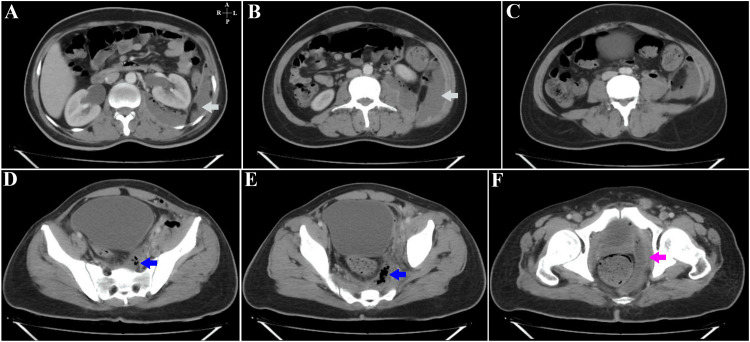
Patient 1- Contrast-enhanced CT images of the abdomen in the axial plane Figure 3A – At the level of the L3-L4 intervertebral disc space, showing a peripherally enhancing collection (cyan arrow) in the posterior, left lateral extra-peritoneal space, extending posterior to the descending colon and the left kidney. Figure 3B – Through the body of the L4 vertebra, an extension of the same collection (cyan arrow) is seen with fascial thickening and abutment of the left transversus abdominis. Figure 3C – At the inferior margin of the L4 vertebral body. Air foci are seen within the collection with subtle stranding of surrounding fat. Figure 3D – At the level of the S2-S3 vertebrae. Air foci (blue arrow) are seen within the collection with stranding of the retro-vesical fat and abutment of the left psoas major muscle. Figure 3E – At the level of the 4th and 5th sacral vertebrae. The air-containing collection (blue arrow) is seen in the left posterior extra-peritoneal rectal space. Thickening of the mesorectal fascia is seen with regional fat stranding. Figure 3F – A peripherally enhancing air-containing collection (pink arrow) is noted in the left hemi-pelvis at the level of the pubic symphysis. The obturator internus is seen laterally, and the rectum is present medial to the collection.
